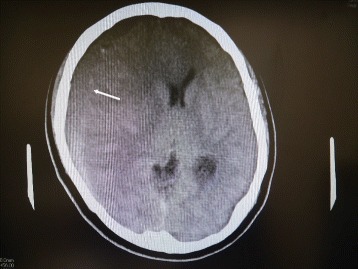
Computed tomogram obtained at the time of readmission to the emergency room. A subdural hematoma (*arrow*) is seen
